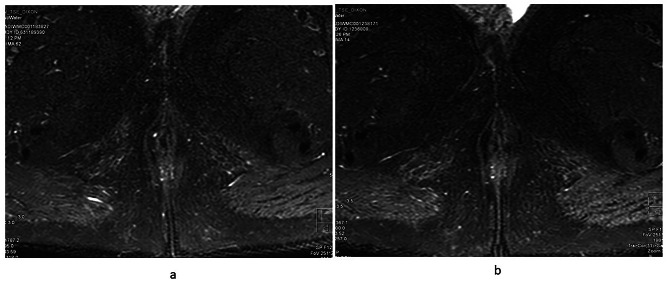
MRI image of a patient in remission (a) At baseline - a linear fistulous tract is seen which is hyperintense on T2W sequence, (b) at 104 weeks – the fistulous tract becomes hypointense on T2W suggestive of fibrosis
